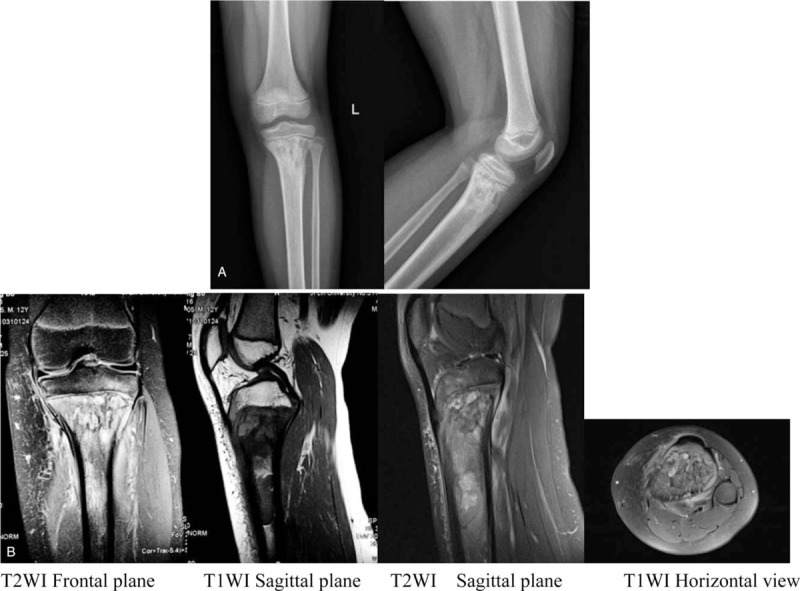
(A) The X-ray of knee showed a permeative lesion in the metaphysis and diaphysis of the left proximal tibia. (B) The MRI of knee demonstrated swelling of the left proximal tibia metaphysis and epiphysis and surrounding soft tissues. The lesion showed hypointensity on T1-weighted imaging, hyperintensity, and iso-intensity on T 2-weighted imaging. Local bone cortical discontinuity and a periosteal reaction can be seen. MRI = magnetic resonance imaging.
